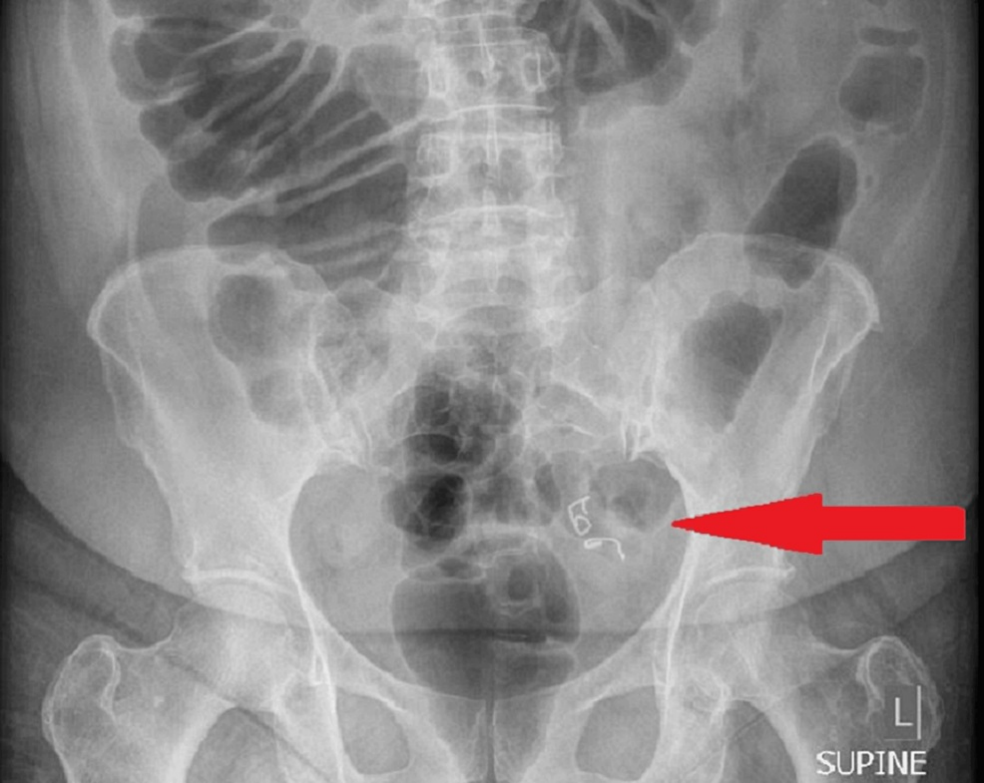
Abdominal X-ray showing the foreign object in the left lower abdomen
